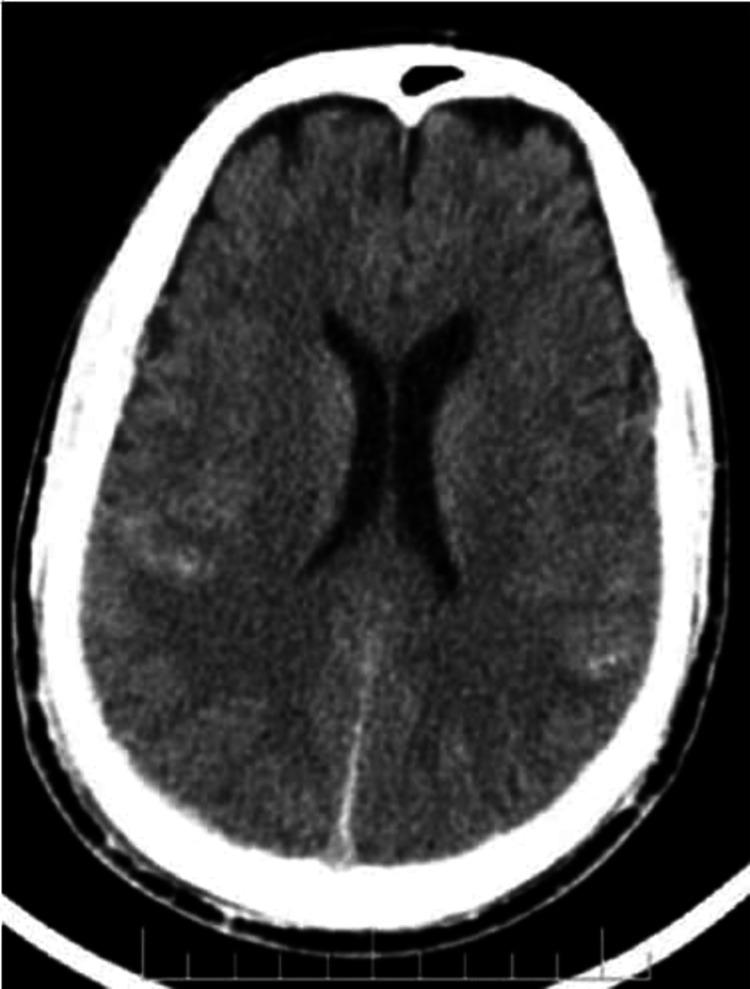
Axial NECT shows a subarachnoid hemorrhage in bilateral parietal regions with no mass effect or compression of basal cisterns - Rotterdam score 2 NECT: non-contrast-enhanced computed tomography
